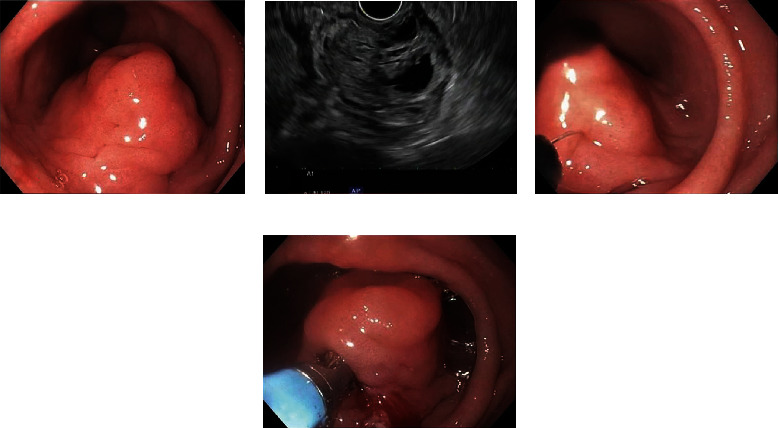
(a) A gastric antral submucosal lesion. (b) Endoscopic ultrasonography shows the origin of the lesions in the muscularis propria. (c) Mucosa incision with needle knife. (d) Biopsy forceps are used for tissue acquisition.
